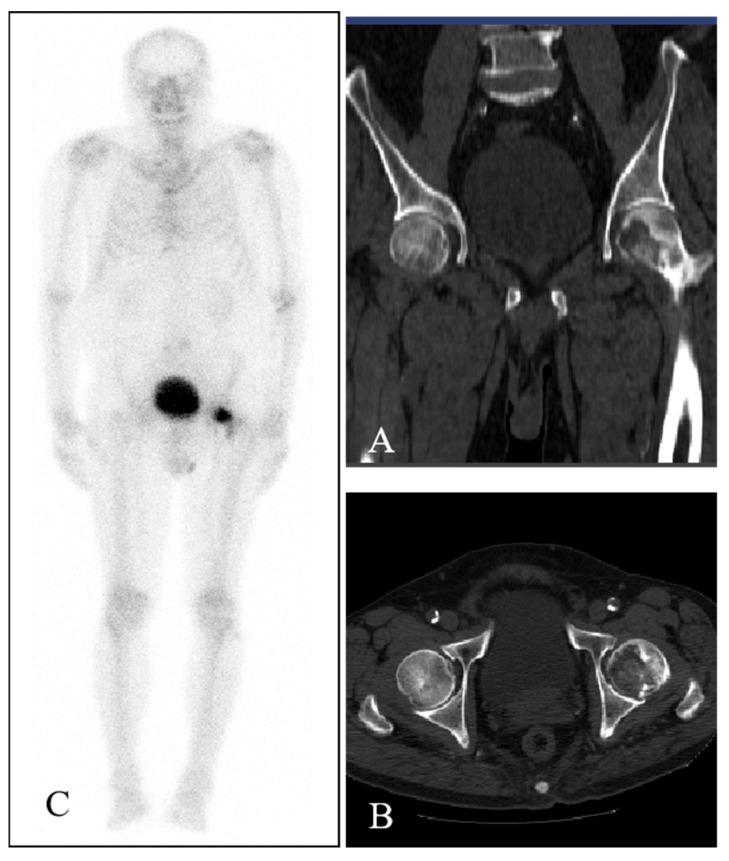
The coronal (A) and axial (B) CT images of bilateral hips showed a low density bone destruction in the left femoral head. The whole-body bone scintigraphy with ^99m^Tc-MDP showed one focal intense area of tracer uptake in the left proximal femoral region (C).
